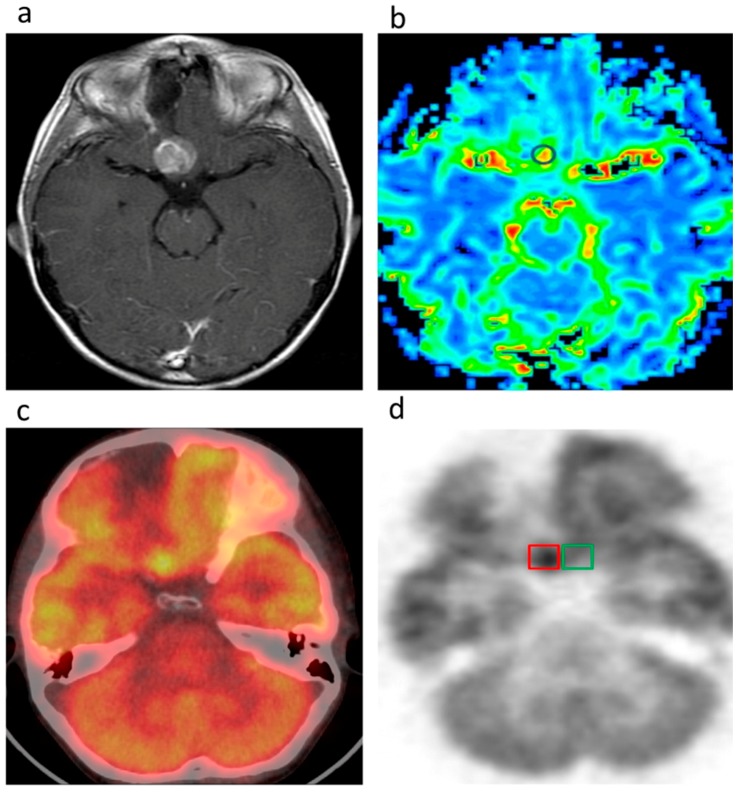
This male patient with a history of right frontal lobe GBM presents with increased enhancement on axial T1-weighted imaging (a) 12.3 months after completion of radiation therapy. The region of interest analyses for the MRI perfusion (b) and FDG PET-CT (c,d) examinations demonstrate an rCBV_max_ of 3.6 and SUV_ratio_ of 2.0. The SUV_lesion max_ is 8.2 (red box), and the SUV_normal brain_ is 4.1 (green box). The lesion was resected and pathologically proven to be recurrent tumor. Adapted with permission from Reference [25].
